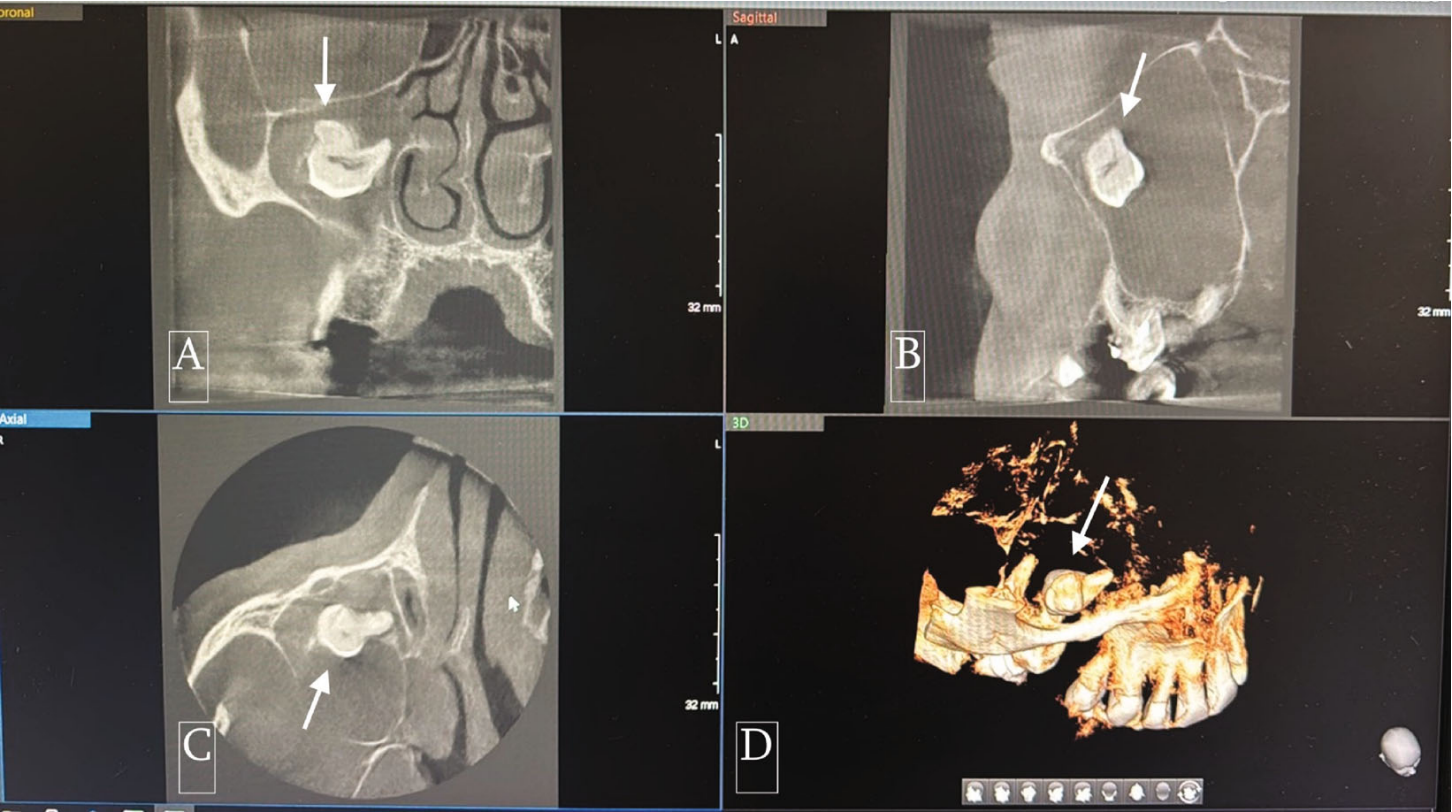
Preoperative cone beam computer tomography image showing ectopic tooth (white arrows) in the right maxillary sinus. (A) Coronal view. (B) Sagittal view. (C) Axial view. (D) 3D reconstruction.
